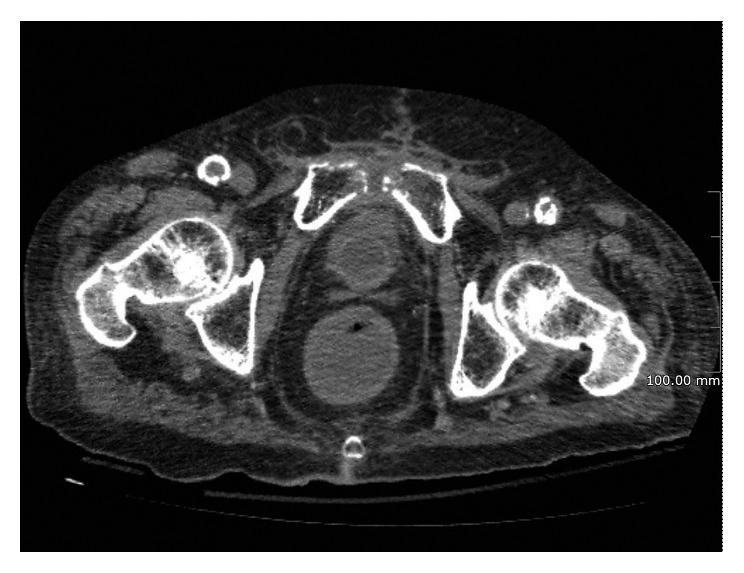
CT scan transverse section showing the erosion of the pubic symphysis surrounded by inflammatory tissue.
